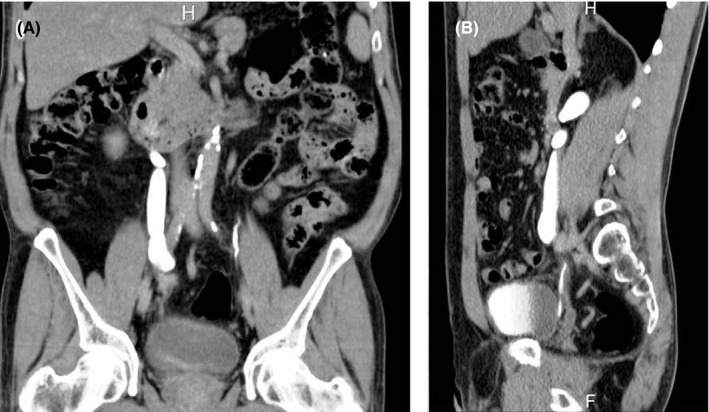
(A, B). Computed tomography (CT‐KUB) (Figure 1) showed the presence of a stricture of 11 mm x 5 mm at the middle third of the ureter at the level of common iliac vessels with dilation of the proximal ureter and renal pelvis. The lumen of the affected ureter appeared to be irregular in shape
